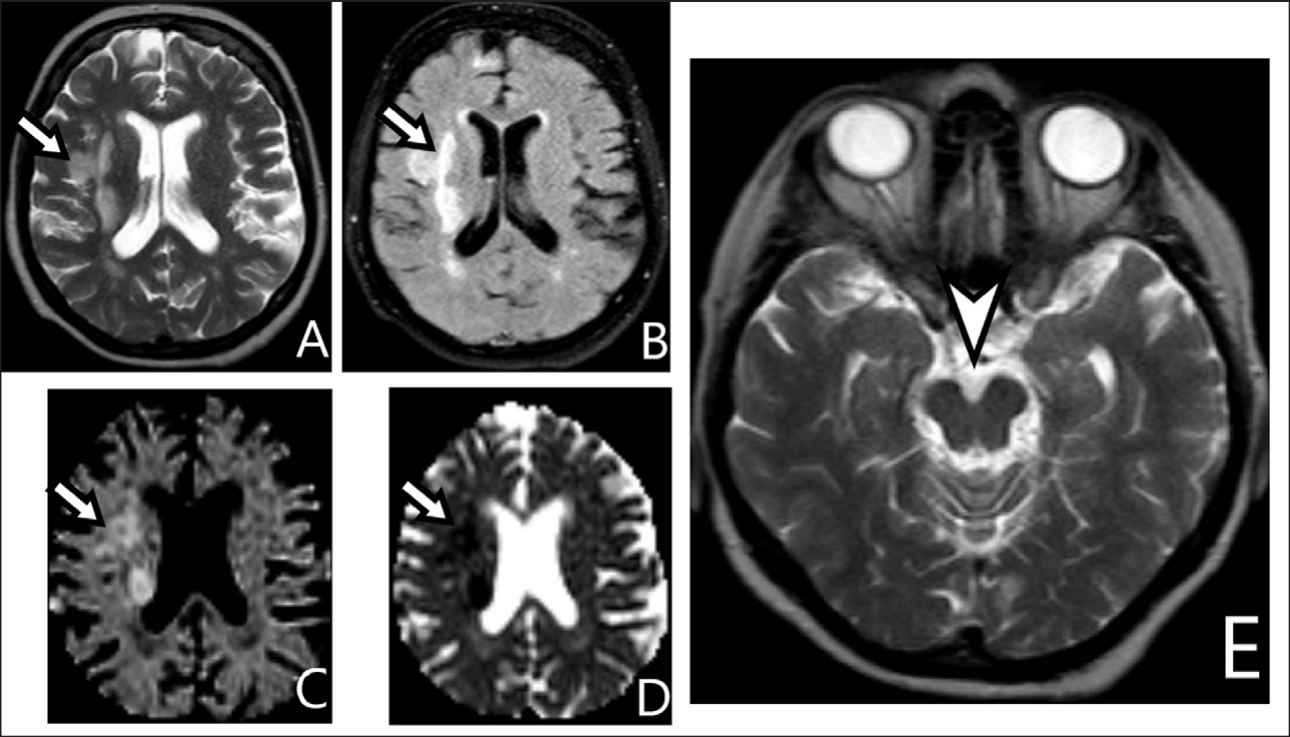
The perfusion area of the right middle cerebral artery is hyperintense on axial T2-weighted Imaging (WI) (A) and Fluid-Attenuated Inversion-Recovery (FLAIR) (B), also hyperintense on Diffusion-Weighted Imaging (DWI) (C) and hypointense on Apparent-Diffusion Coefficient (ADC) map (D) (*arrows*). The cerebral peduncles are symmetric on T2-WI (E), (*arrowhead*).
